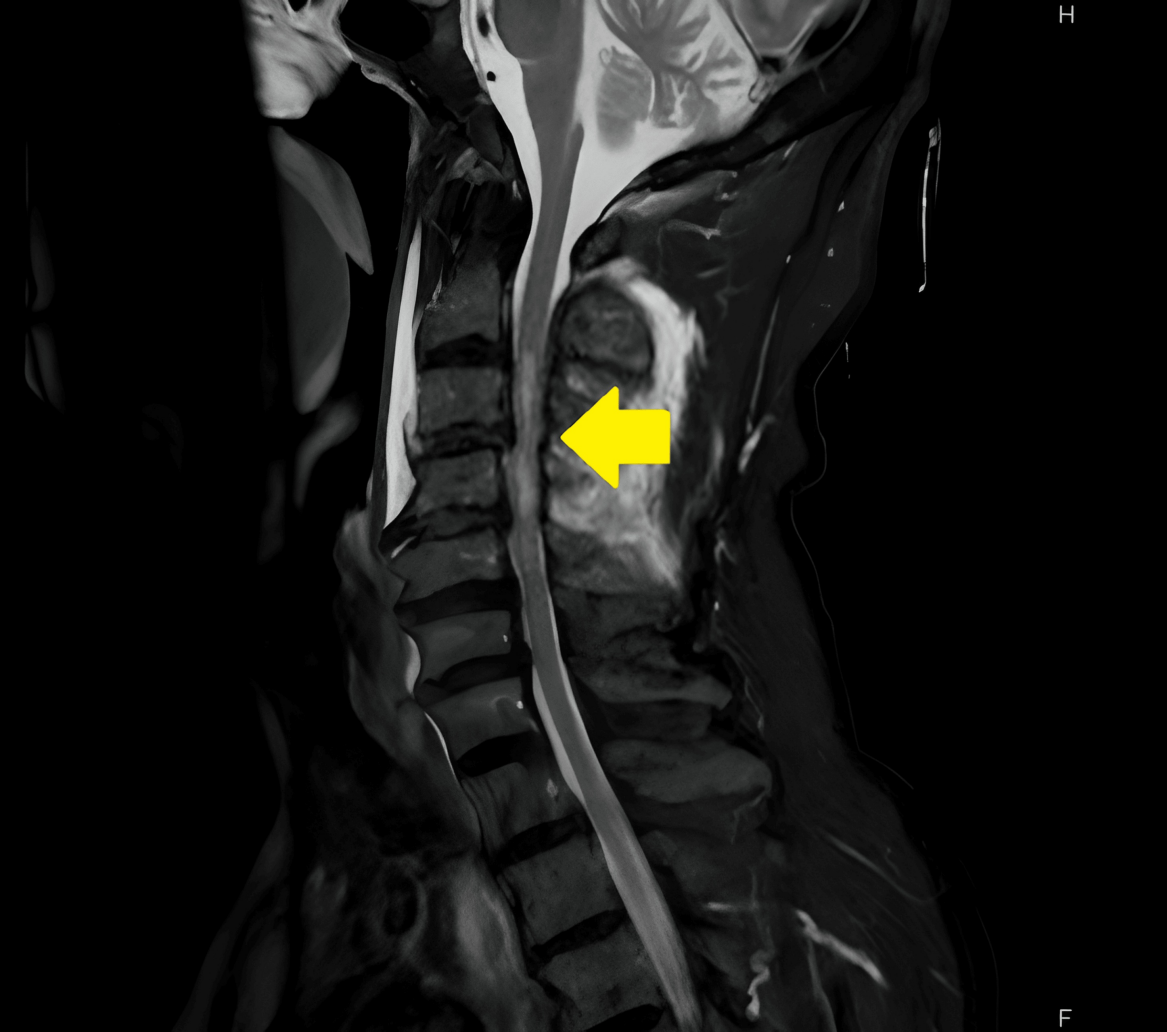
STIR phase of MRI High-intensity signal at T3-4 with no abnormal findings in the cervical vertebrae. STIR, short T1 inversion recovery
